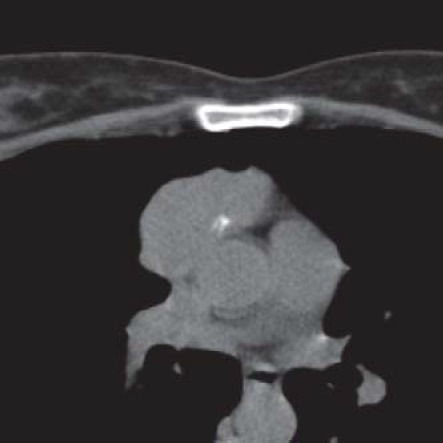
35-y/o female with myasthenia gravis; CT scan shows a tumoral mass in the prevascular space with minimal calcification; pathologic diagnosis - thymoma
